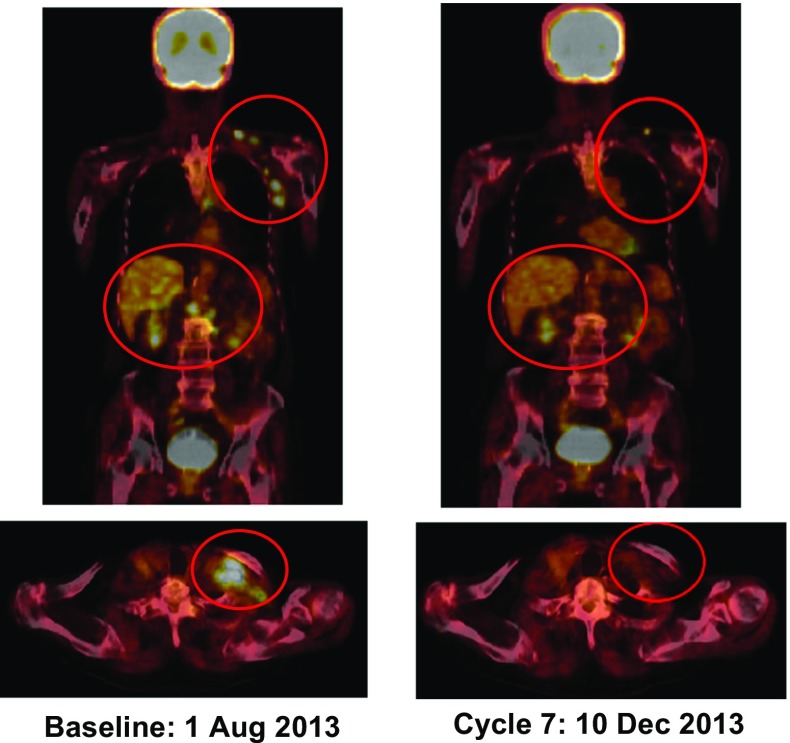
PET-CT images taken before the start of treatment and after cycle 7 show evidence of antitumor activity. A rapid and durable partial response according to irRC was observed in a 91-year-old man with advanced metastatic acral lentiginous melanoma who had active disease in the liver and multiple lymph nodes when treatment with pembrolizumab at 10 mg/kg Q2W was initiated
